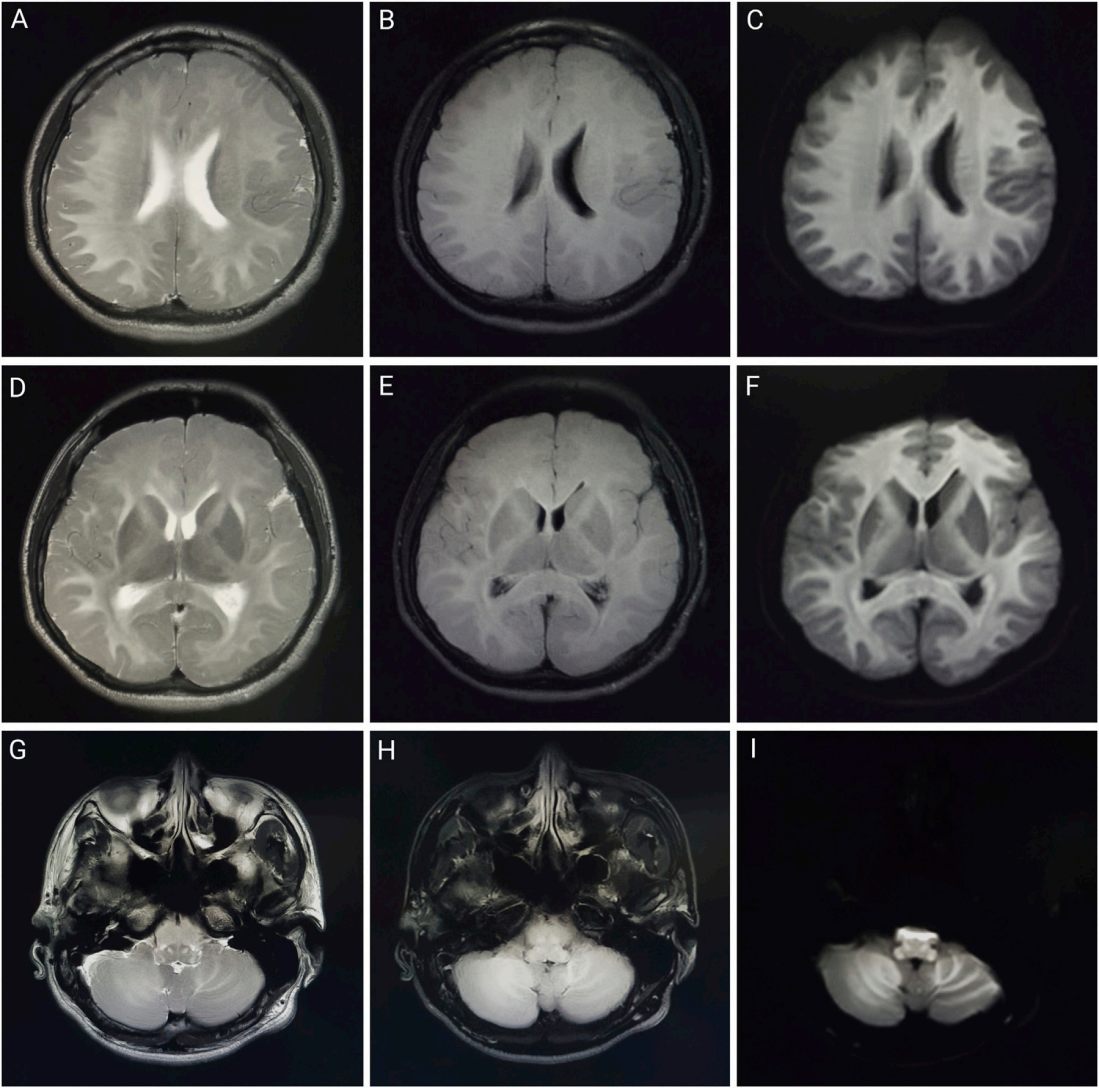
Brain MRI of patient one on day 1 of admission. Brain magnetic resonance imaging of a 23-year-old man after working in a workshop for 3 weeks. Abnormalities included diffuse symmetrical abnormal signals in the bilateral cerebral hemispheres, bilateral cerebellar hemispheres, and brainstem. (A,D,G) are T2-weighted images; (B,E,H) are T2 fluid-attenuated inversion recovery (FLAIR) images; (C,F,I) are diffusion-weighted images.
